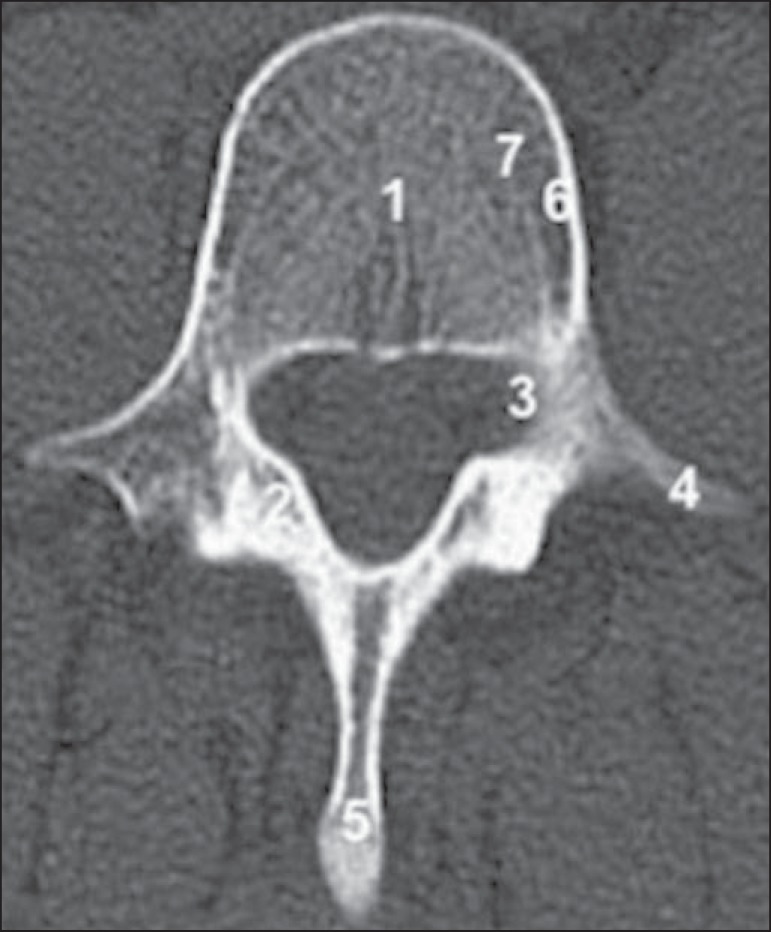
Computed tomography (cross section) of the backbone, L1 level, showing
